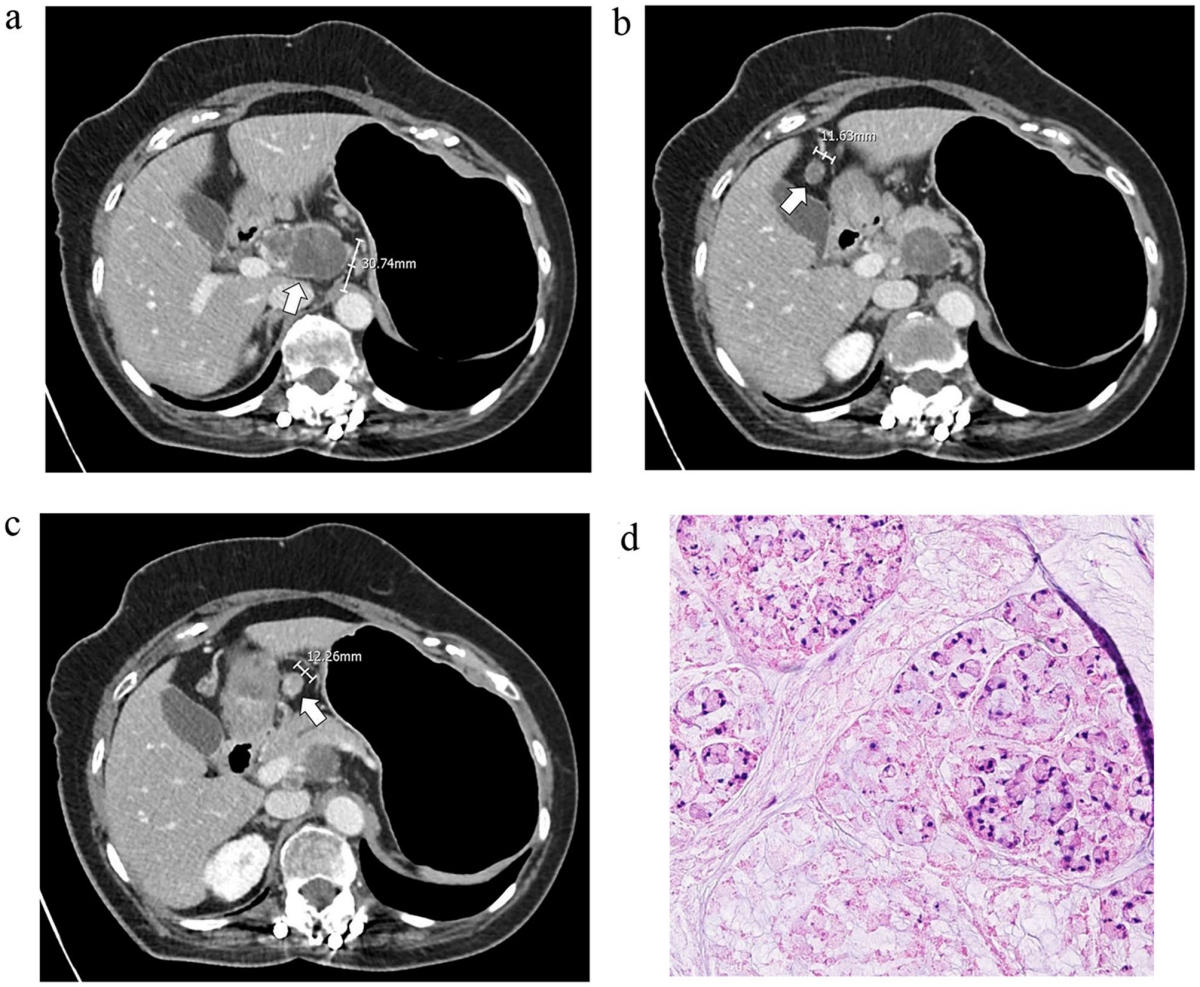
A 65 year-old female patient diagnosed with advanced gastric cancer. Preoperative CT imaging revealed lymph nodes measuring up to 30 mm in the common hepatic station, as well as 12 mm and 11 mm lymph nodes in the supra- and infrapyloric stations, respectively (a-c). Pathological examination confirmed these lymph nodes to be metastatic mucinous carcinoma (d)
